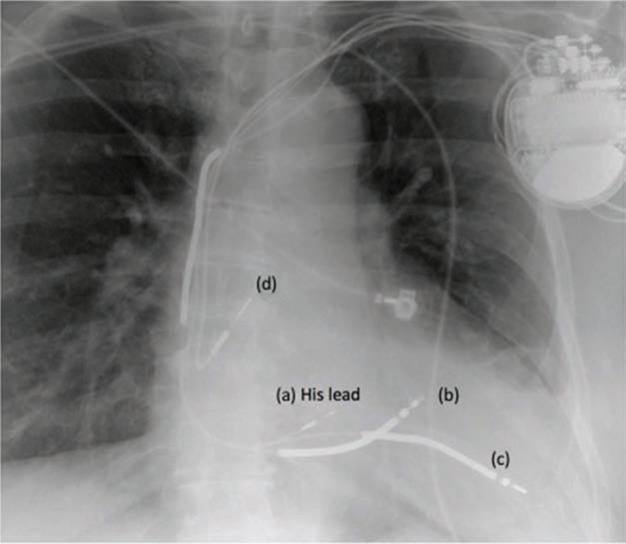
A chest X-ray taken post-procedure. (a) His-bundle pacing lead. (b) The new high voltage lead in the right ventricle. (c) The old high voltage lead in the middle cardiac vein. (d) A pacing lead in the right atrium.
